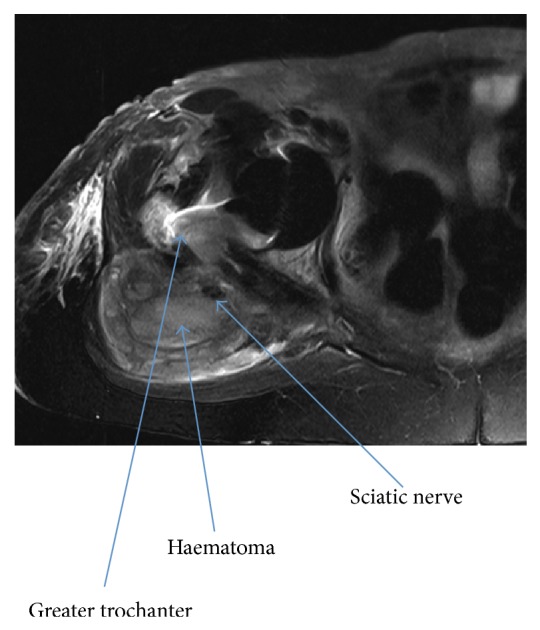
Axial T2 MRI scan showing the haematoma compressing the sciatic nerve.
